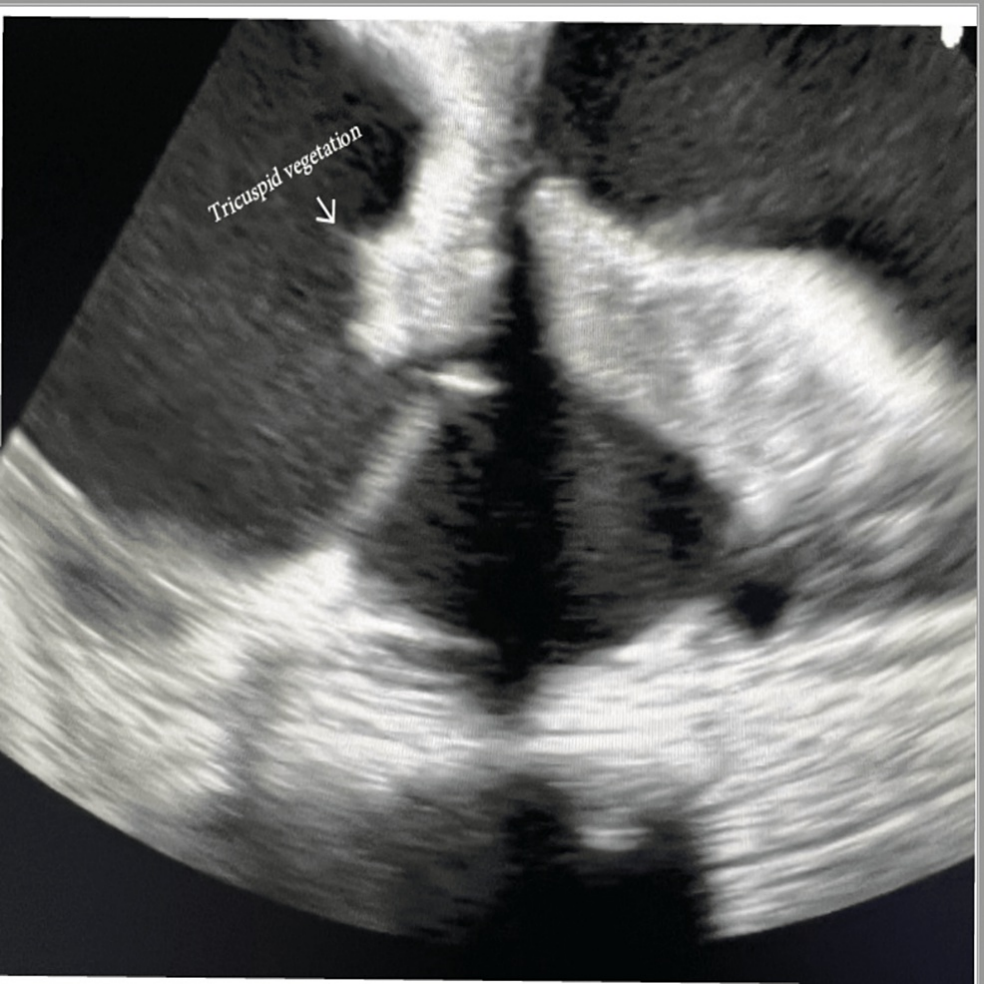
Tricuspid vegetation observed on transesophageal echocardiogram.
